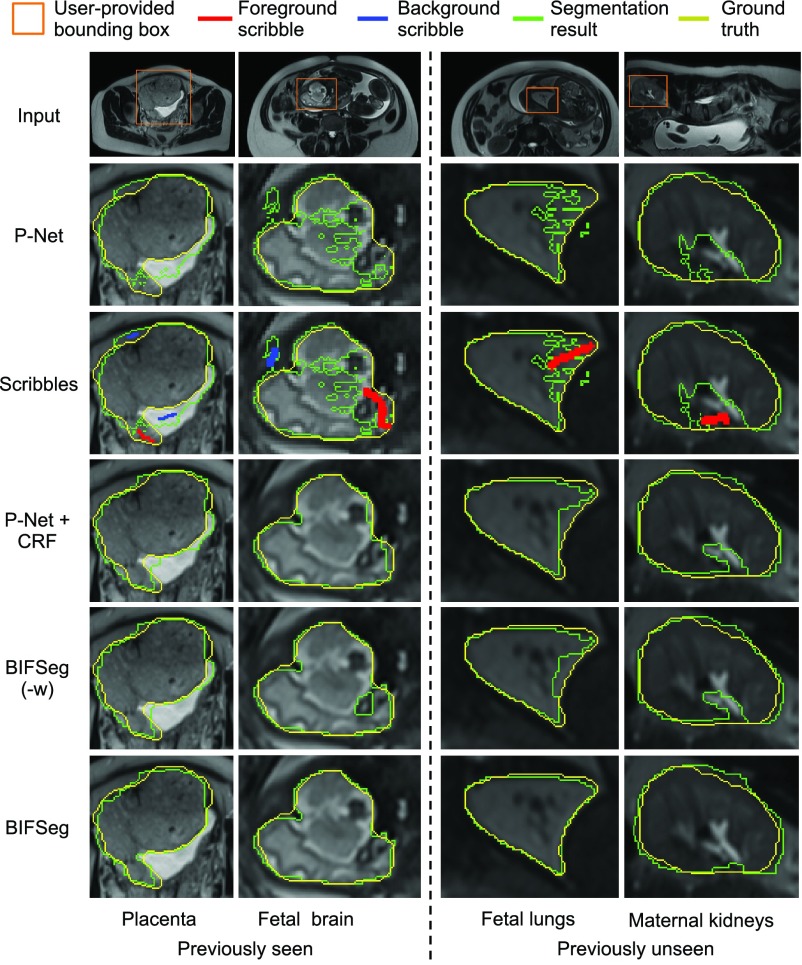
Visual comparison of P-Net and three supervised refinement methods for fetal MRI segmentation. The same initial segmentation and scribbles are used for P-Net + CRF, BIFSeg(-w) and BIFSeg.
